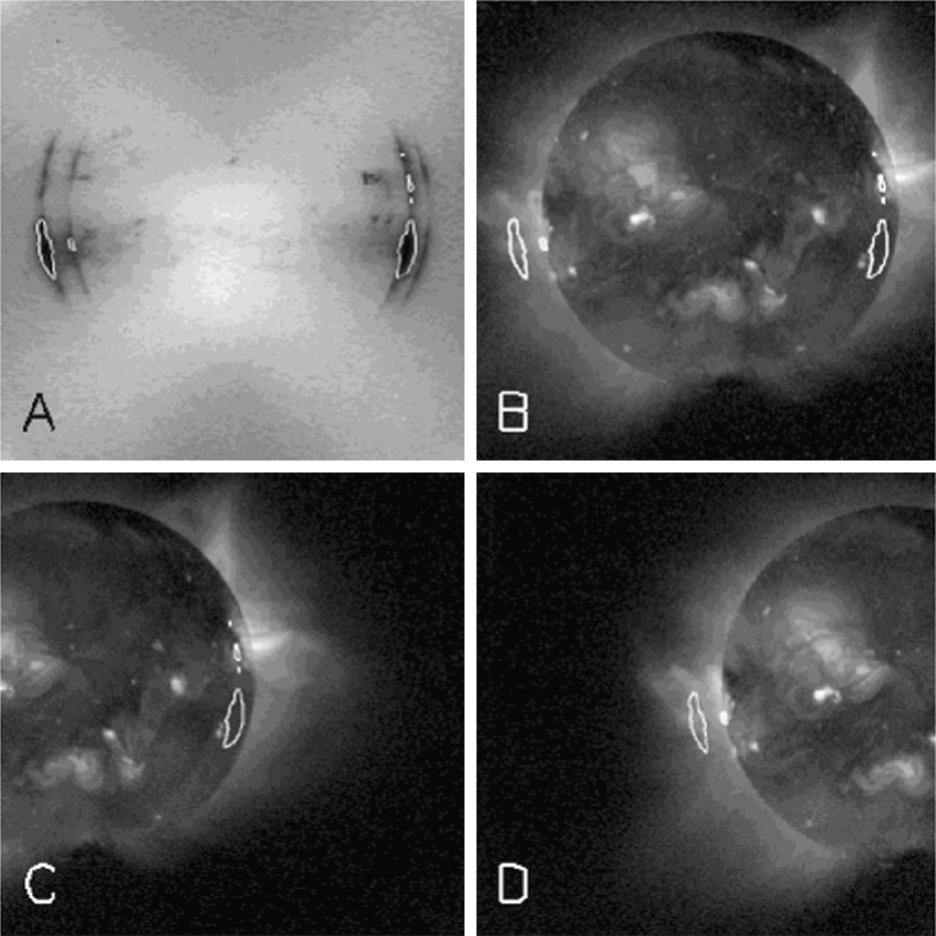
Sample areas for test of SXT X-ray quantum efficiency in optical burn areas. (A) diffuser image of 26 August 1992, 19:25 UT. Contours denote areas of strongest ionizing radiation damage (burns). (B) log-scaled X-ray image (Al.1 filter) obtained at 19:50 UT with burn contours. (C) X-ray image taken at 21:25 UT during a *Yohkoh* offpoint to the west. (D) X-ray image of 22:58 UT during offpoint to the east. Burn contours in the X-ray images show the image areas sampled for the signal level plots of Figure 52.
